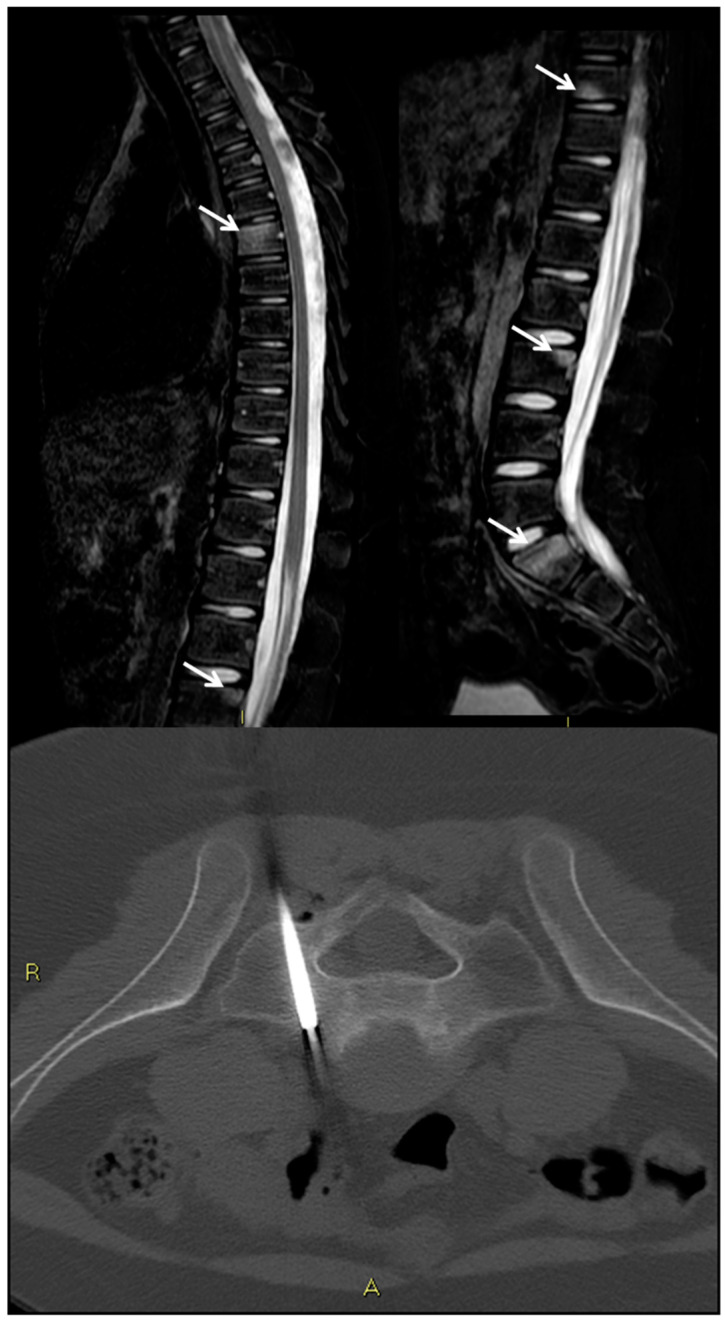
Chronic recurrent multifocal osteomyelitis (CRMO) in an 11-year-old boy. MRI shows several areas of bone edema in thoracic, lumbar, and sacral vertebral bodies (arrows). After a CT-guided bone biopsy of S1, the diagnosis of exclusion was CRMO.
